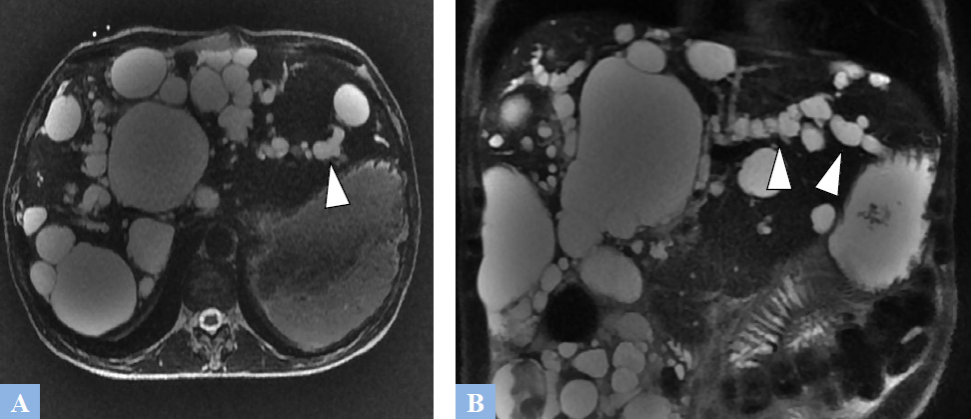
PLD in a 54-year-old patient presenting with non-febrile jaundice, leading to the indication for liver transplantation. A. T2-weighted fat-suppressed MR image in the axial plane showing hepatic polycystic disease with multiple peri-hilar cysts compressing the hepatic hilum, associated with upstream biliary duct dilation (arrowhead). B. T2-weighted fat-suppressed MR image in the coronal plane. Dilation of the intrahepatic bile ducts of the left lobe (arrowhead).
